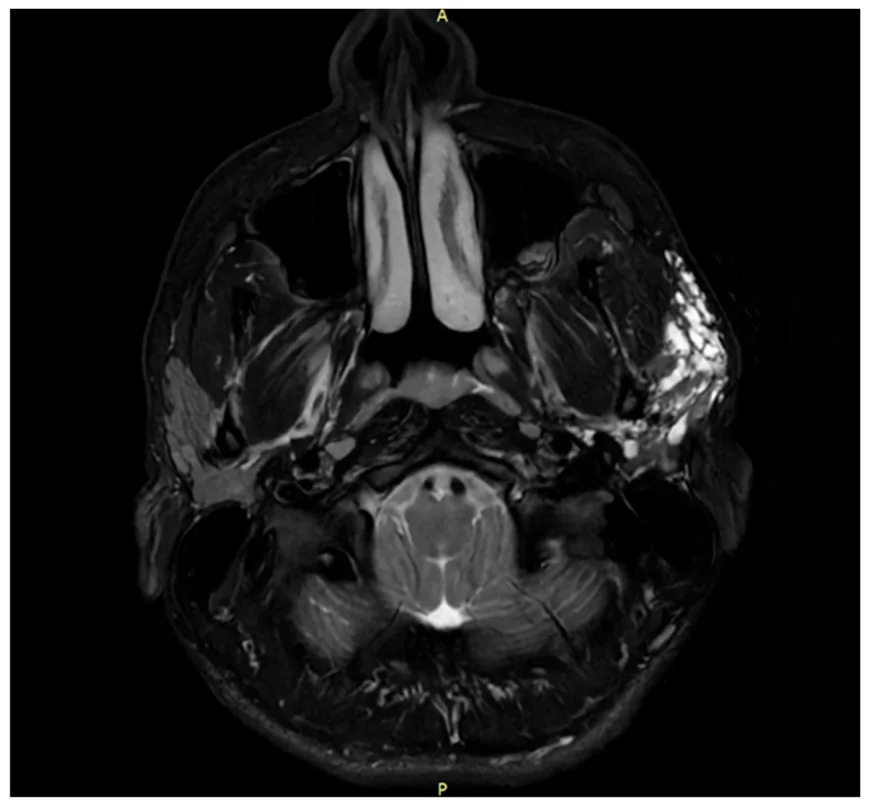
Axial T2-weighted fat-saturated magnetic resonance image before treatment. An extensive lymphatic malformation occupies the left parotideo-masseteric region and the parotid gland, showing irregular walls and high signal content, and extending medially towards the parapharyngeal space.
